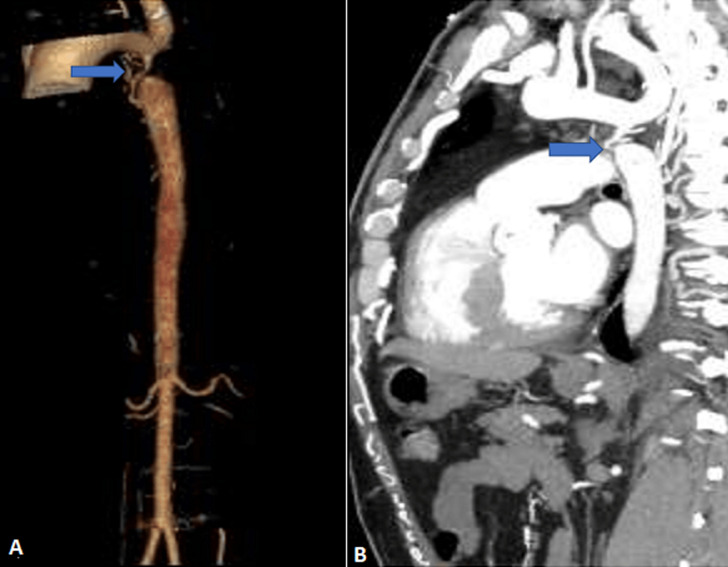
volume rendering A) and sagittal B) reconstruction of aortic CT images, showing a very tight narrowing of 2cm downstream of the origin of the supraclavicular artery (arrow)
